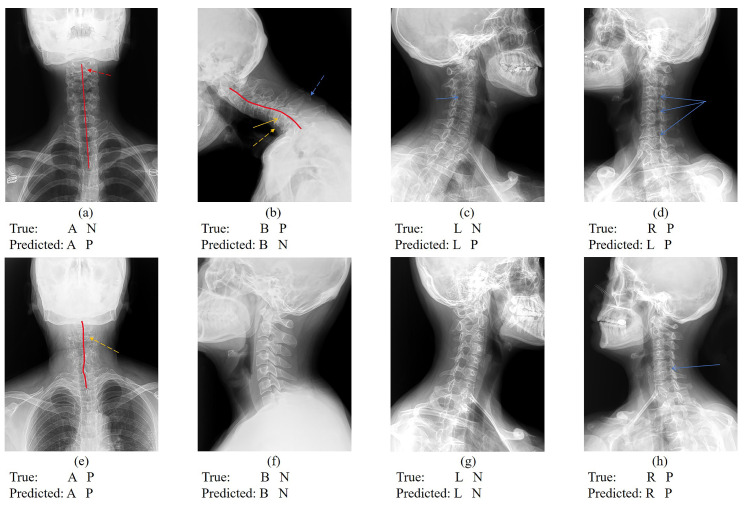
Cervical vertebra X-ray projection position classification and CS classification results. Figure (a–c) represent that the cervical spine position is correctly classified but CS is incorrectly classified. Figure (d) represents that the cervical spine position is classified incorrectly but CS is correctly classified. Figure (e–h) represent images with correct cervical vertebra position classification and CS classification. (“N” indicates CS is negative; “P” indicates CS is positive; the red line indicates the physiological curvature of the cervical spine; the red dotted arrow indicates the hyoid bone blocking the cervical vertebrae. the yellow arrow indicates the intervertebral space; the yellow dotted arrow indicates osteophyte formation; the blue dotted arrow indicates ligament calcification; and the blue arrow indicates the intervertebral foramen).
